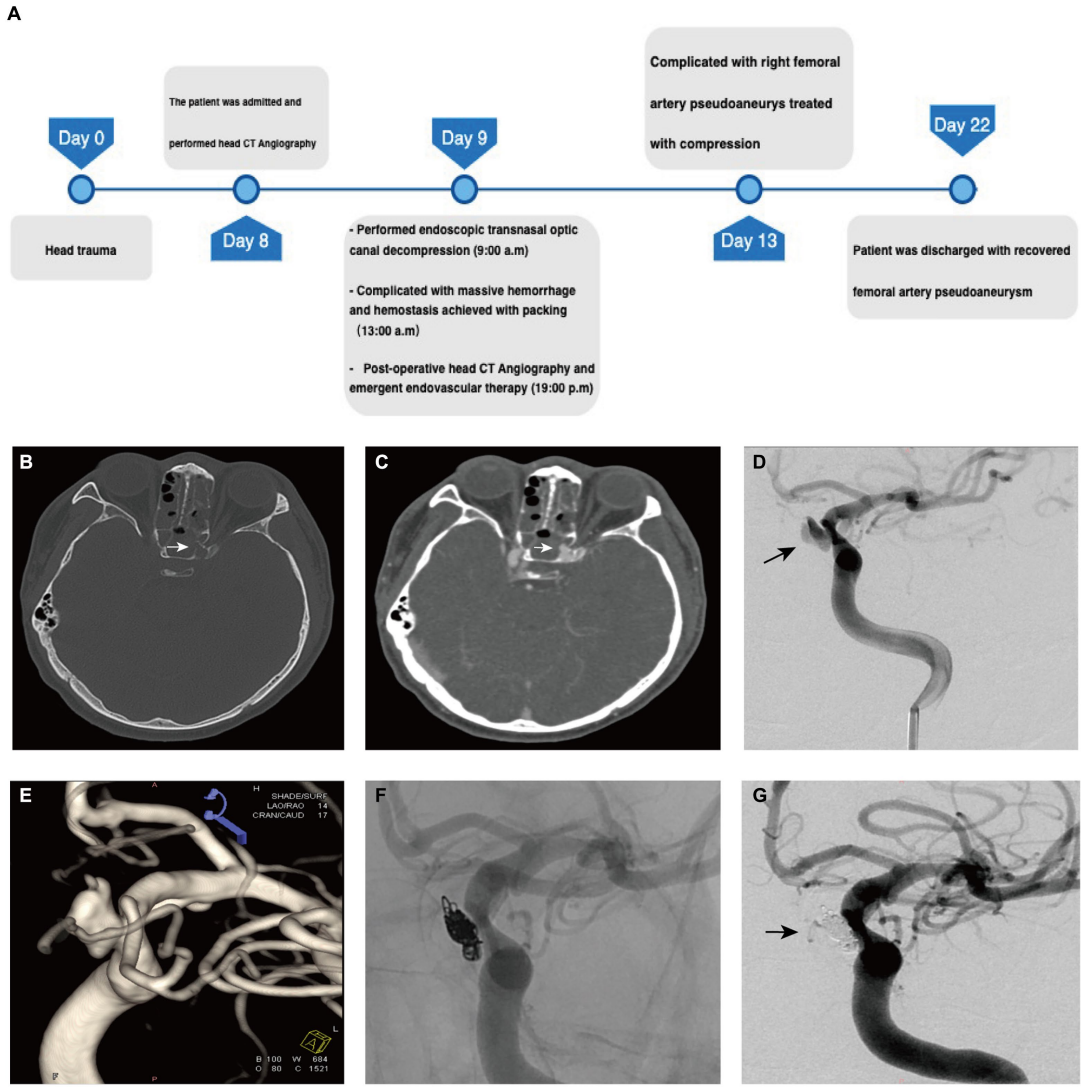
The preoperative evaluation and surgical process in Case 2. (A) The timeline of management in case 2. (B) Preoperative CT of the head demonstrates lateral wall of sphenoid sinus cavity in the left that protrudes into the optic canal (*arrow*). (C) Postoperative CTA shows enhancement of the central patent portion of the pseudoaneurysm that protrudes into the sphenoid sinus cavity (*arrow*). (D) The left internal carotid injection of the contrast demonstrates a pseudoaneurysm arising from ICA. (E) 3D reconstruction of DSA demonstrates the irregular pseudoaneurysm with the feature of re-rupture (*arrow*). (F) The angiogram obtained after endovascular treatment demonstrates complete occlusion of the pseudoaneurysm with coils. (G) Post-embolization angiogram demonstrates complete occlusion of the pseudoaneurysm cavity (*arrow*).
